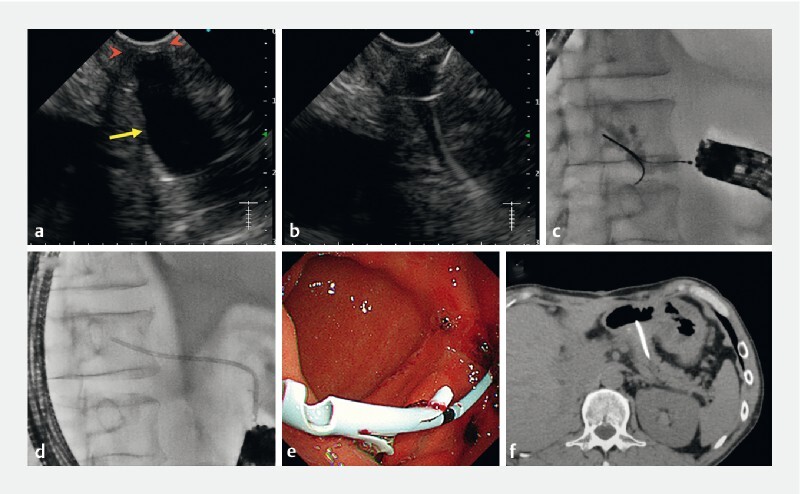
Second attempt at endoscopic ultrasound (EUS)-guided re-pancreaticogastrostomy using a forward-viewing echoendoscope.
